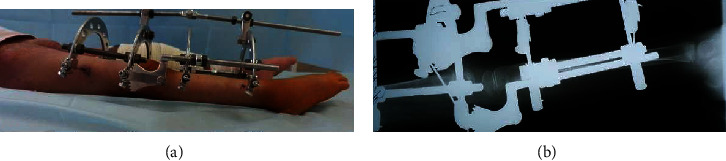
(a, b) Correction of knee joint deformation was achieved (a). Full extension of the knees has been achieved. Note that the lateral radiograph showed the full correction of the knee contractures (b).
